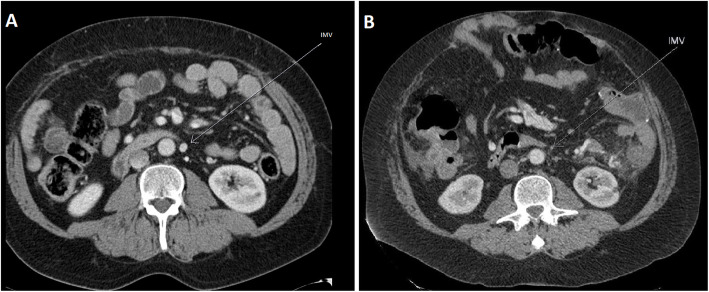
Contrast enhanced CT abdomen, portal venous phase. Demonstrating patent IMV at baseline (image a, Arrow) which had narrowed 7 years later (image b, Arrow). The patient underwent his first sclerotherapy treatment after image b was acquired.
